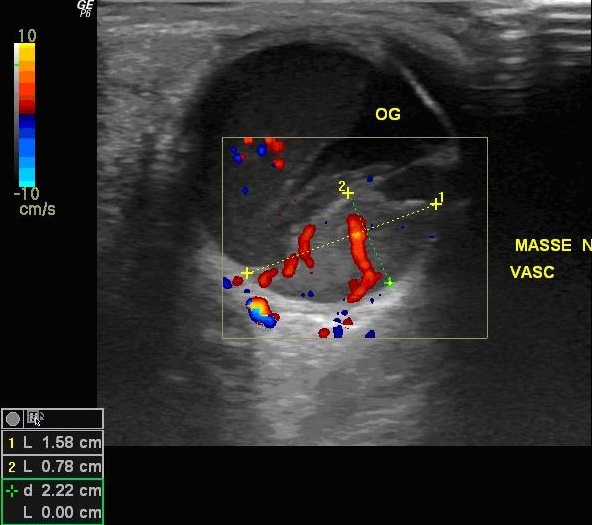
Échographie oculaire en mode B couplée au doppler montre une volumineuse masse nasale 15mm/ 7,8 mm, à surface irrégulière à contenu tissulaire hétérogène présentant une vascularisation centrale évidente au doppler avec soulèvement rétinien et épanchement sous rétinien
